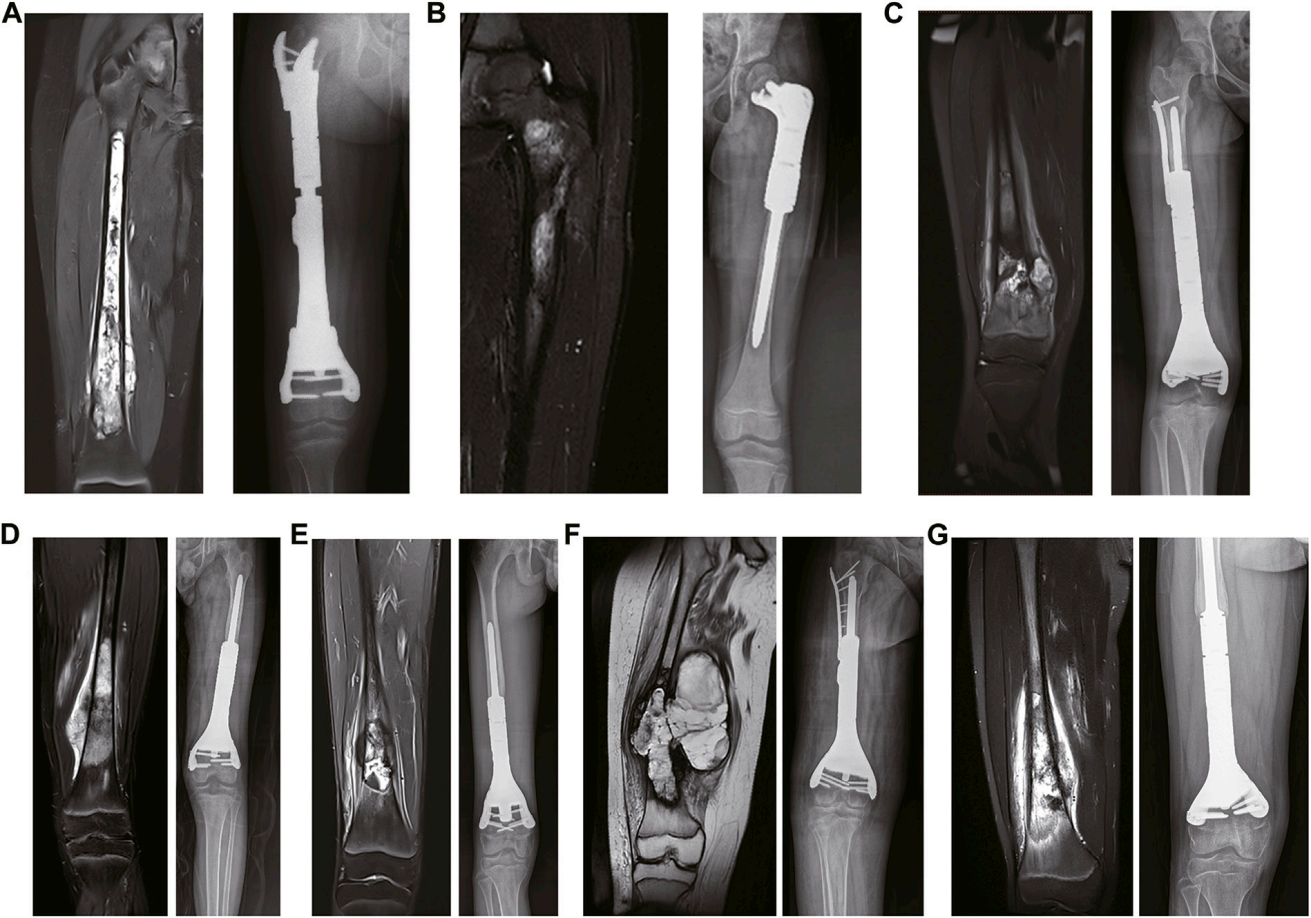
The preoperative and postoperative MRI results. (A) The preoperative coronal MRI results of case 1. (B) The preoperative coronal MRI results of case 2. (C) The preoperative coronal MRI results of case 3. (D) The preoperative coronal MRI results of case 4. (E) The preoperative coronal MRI results of case 5. (F) The preoperative coronal MRI results of case 6. (G) The preoperative coronal MRI results of case 7.
